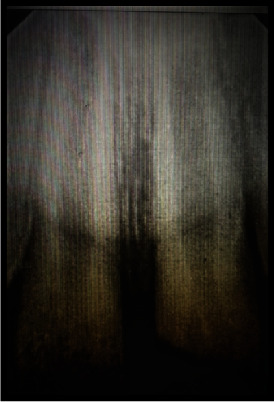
Pre-operative radiographs of teeth #11 and #21 with Cvek's stage 3.
